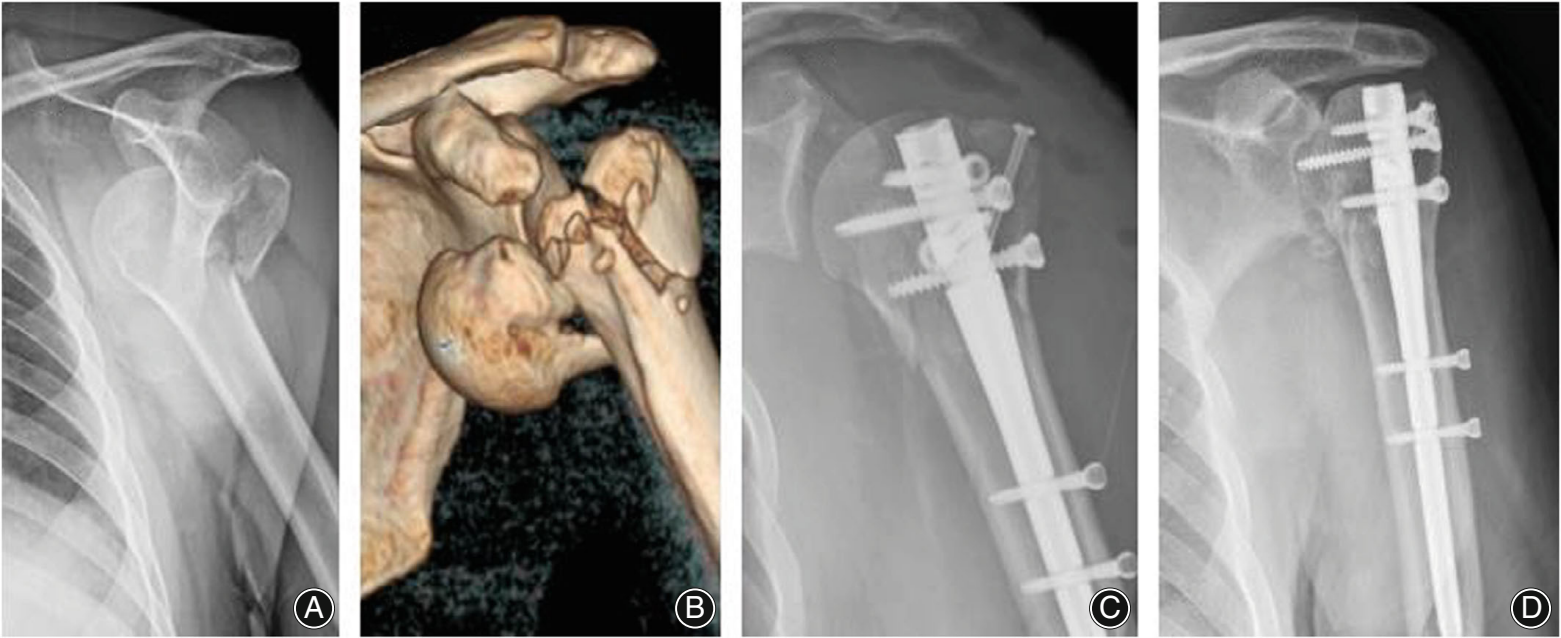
(A) Dislocation and greater tuberosity fracture; (B) Fracture of greater tuberosity and humeral head; (C) Radiograph immediate postoperatively; (D) Radiograph 8 months postoperatively indicating osteonecrosis of the humeral head
